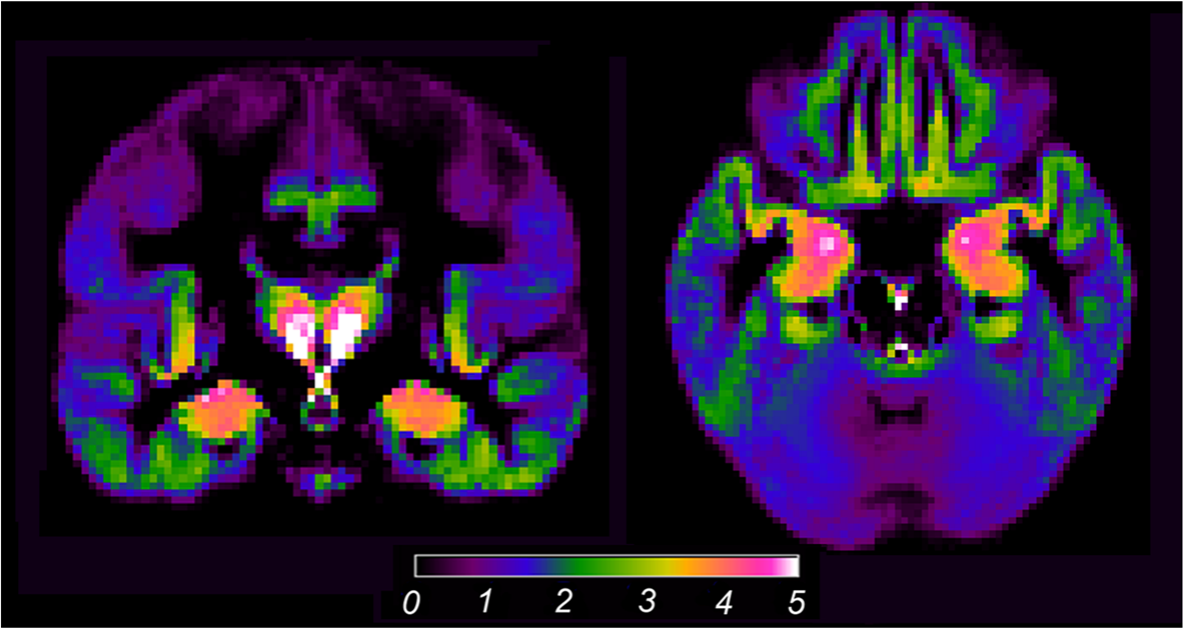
Mean SUVR images of ^18^F-THK5351 in cognitively normal older adults. Localized ^18^F-THK5351 accumulation was mainly evident in the basal ganglia, thalamus, and medial temporal lobe, which slightly extended to the inferior temporal lobe, insula, posterior cingulate gyrus/precuneus, and basal frontal lobe. SUVR, standardized uptake value ratio
